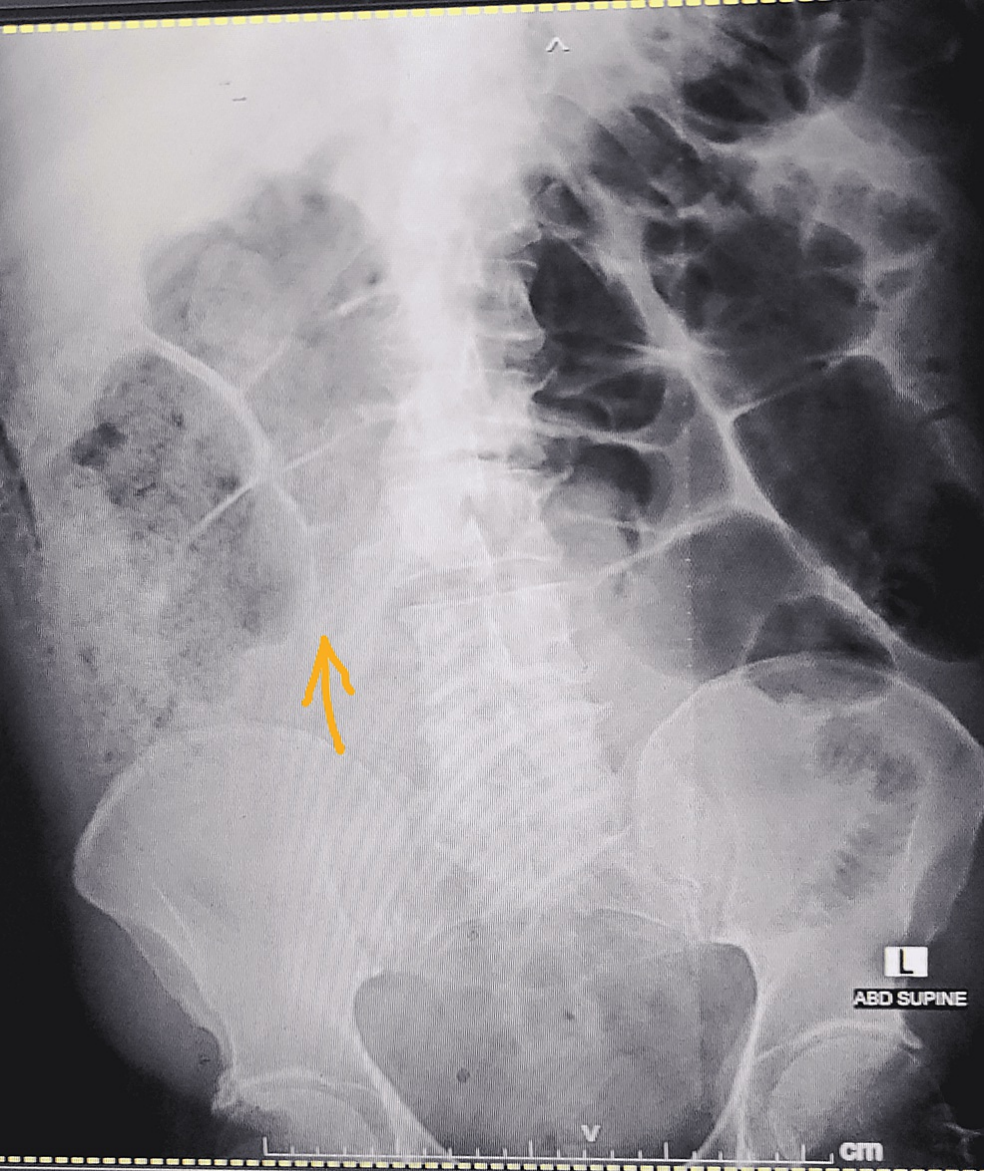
X-ray of the erect abdomen shows pneumoperitoneum
